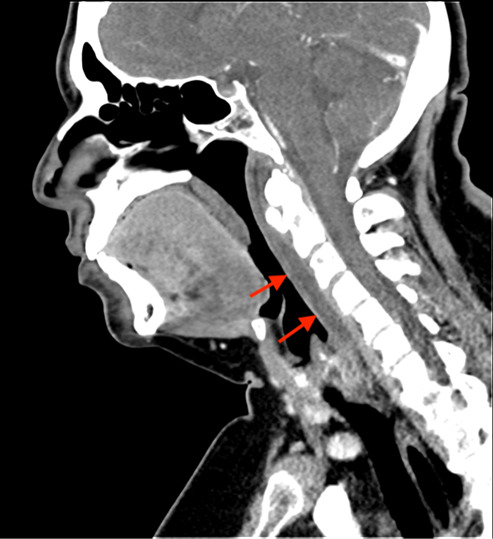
Sagittal computed tomography image in soft tissue window showing diffuse retropharyngeal edema (arrows).
